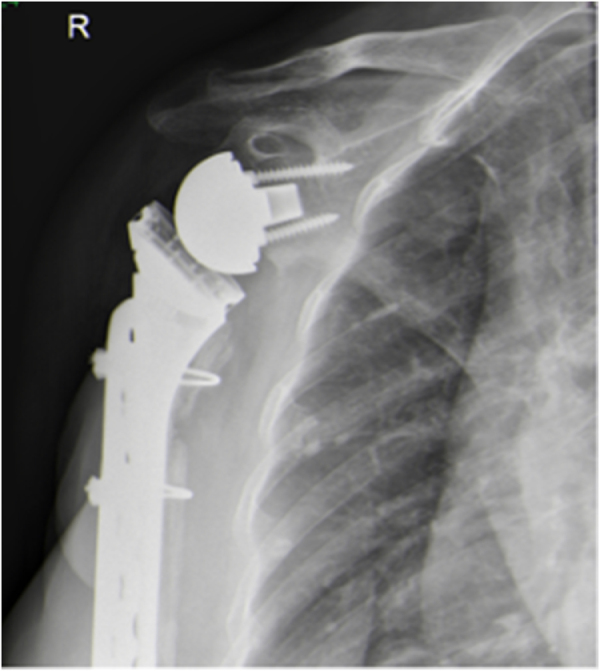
Re-revision postoperative X-ray.
